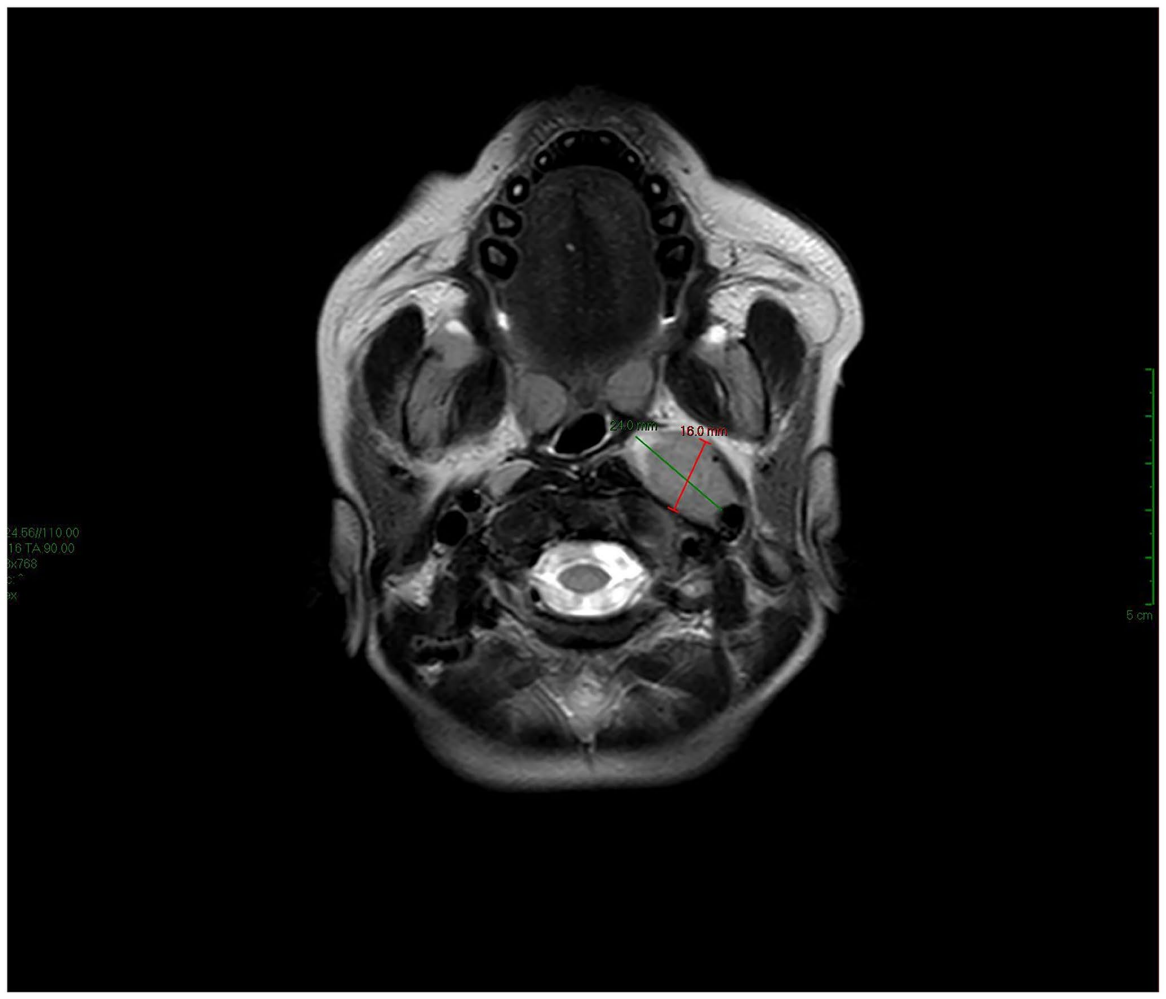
Brain MRI. Prominent cervical lymphadenopathy was noted, with the largest node measuring 24 × 16 mm.
